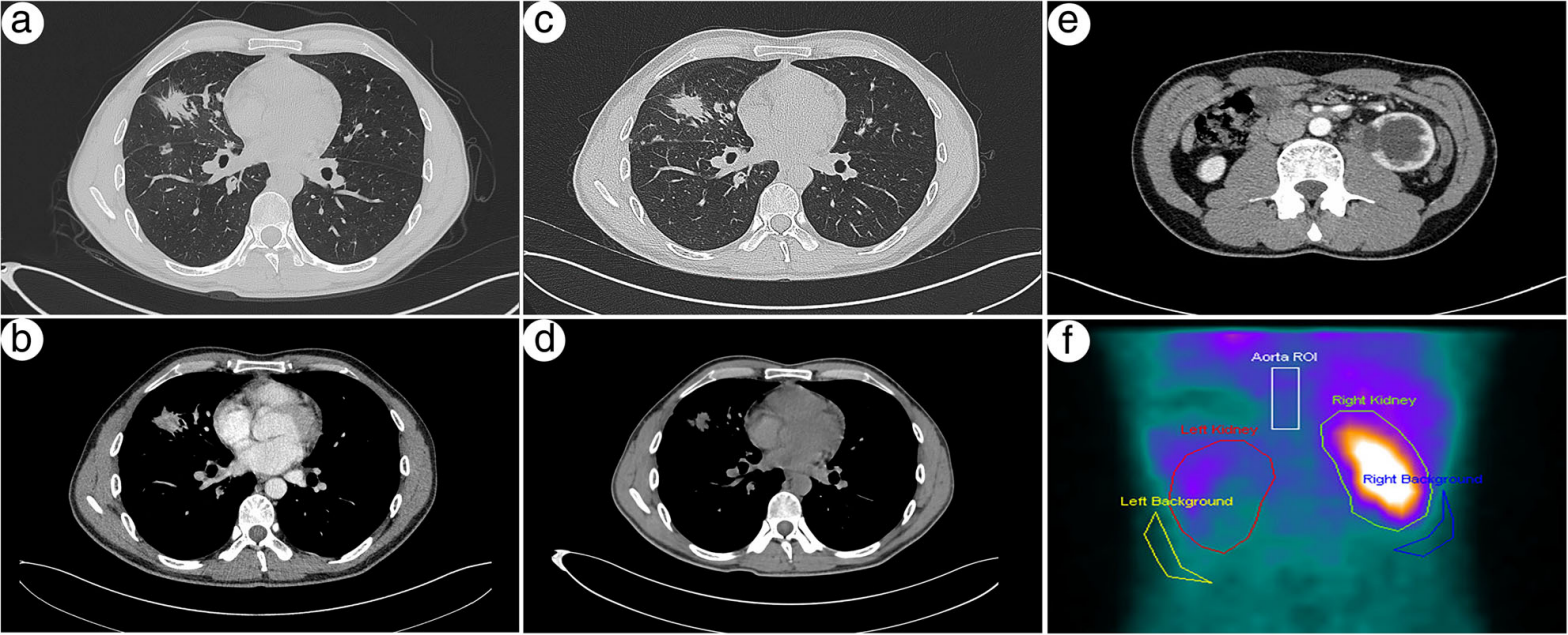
(a,b) Before targeted therapy, chest computed tomography (CT) images revealed a 3.0 cm × 2.4 cm irregular soft tissue mass with heterogeneous enhancement located in the lateral segment of the right middle lobe. (c,d) After 8‐month targeted therapy, chest CT images showed a 2.6 cm × 2.3 cm irregular soft tissue mass. The tumor volume decreased when compared to prior treatment. (e) Abdominal CT images revealed enlargement of the left kidney, a soft tissue nodule with heterogeneous enhancement at the proximal end of the ureter, dilation and hydronephrosis of the left renal pelvis and calyces, thinning of the renal parenchyma, hydronephrosis of the left kidney, and an unclear boundary between the lesion and the left psoas major muscle. (f) SPECT renal dynamic imaging revealed that the perfusion of the left kidney decreased, the shape blurred, and the right kidney was normal
